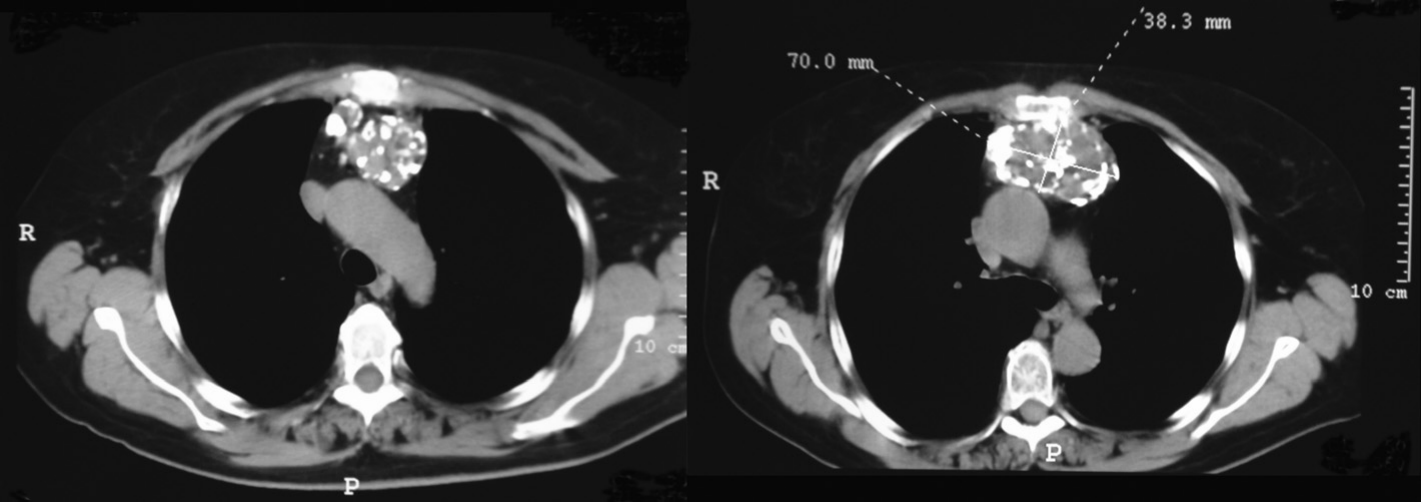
CT images show the mass into the anterior mediastinum. On the right it is possible to observe the calcified connection between the mass and the sternum; no cortical erosion is present.
